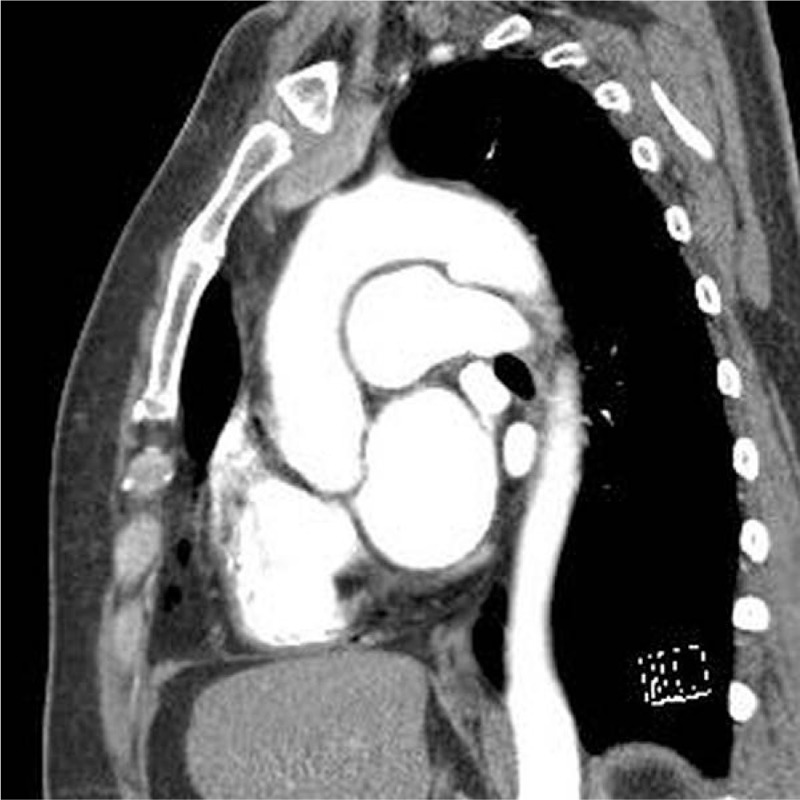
Preoperative three-dimensional computed tomography image of the aorta. Sagittal view showing communication between the aorta and pulmonary artery.
